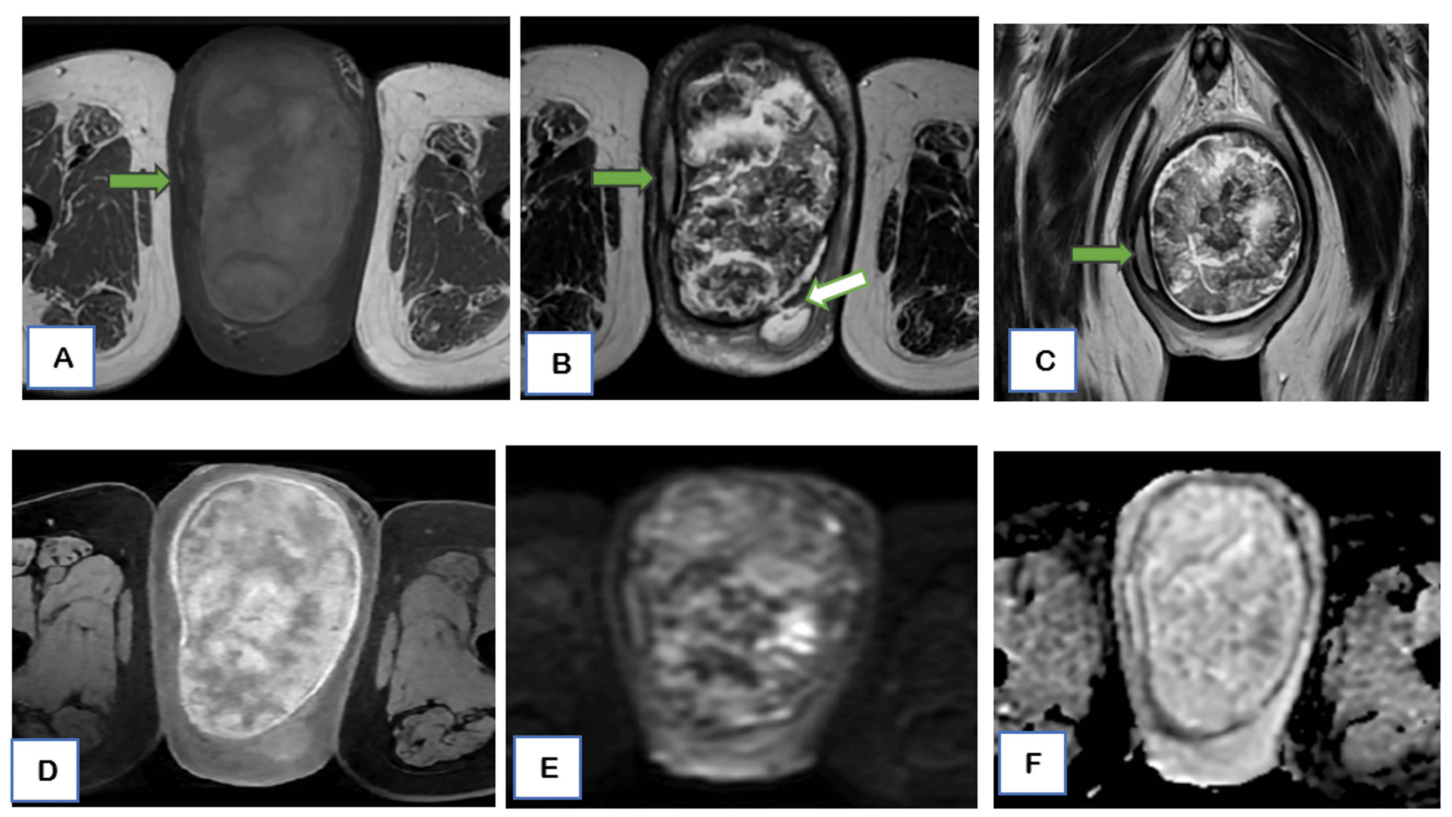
Scrotal MRI images A large, well-defined, encapsulated heterogeneous lesion was seen, which showed solid-cystic components on both axial T1WI (A) and T2WI (B, C) sequences. The left testis is seen compressed and displaced laterally to the right, with maintained fat planes (green arrow). (B) On axial T2WI image, a small capsular focal defect was seen with fluid seen leaking and forming a small collection in the scrotal wall (white arrow).  (D) On dynamic post-contrast axial images, early avid heterogeneous enhancement of the solid areas, with internal non-enhancing regions corresponding to cystic or degenerative components was noted. (E) diffusion weighted imaging (DWI) and (F) apparent diffusion coefficient (ADC) – revealed few small areas of mild diffusion restriction within solid components.
